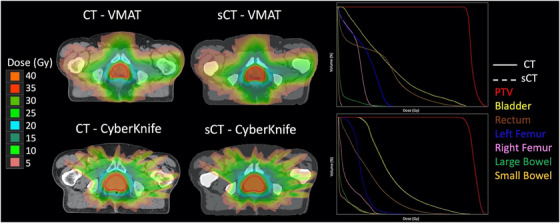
Representative axial dose distributions for VMAT (top row) and CyberKnife (bottom row) plans calculated on a CT (left images) versus sCT (right images). DVH curves in each plot (right column) directly overlay one another, demonstrating close agreement between distributions for sCT versus CT when the optimized sCT calibration curve is used. CT, computed tomography; DVH, dose‐volume‐histogram; sCT, synthetic CT; VMAT, volumetric modulated arc therapy.
